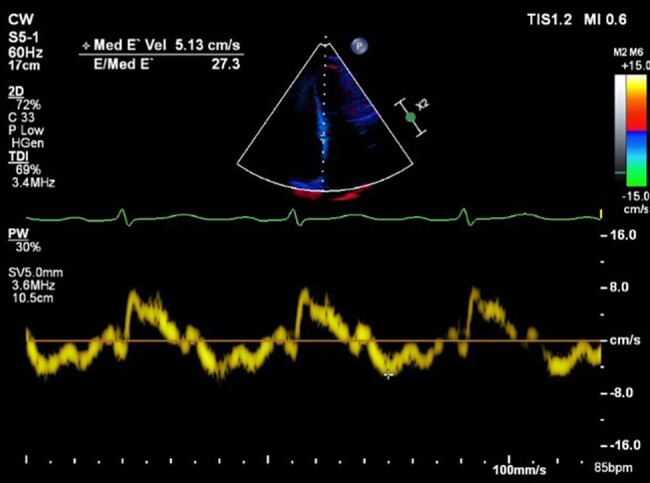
Abnormal medial E/e′ velocities (27.3) (tissue Doppler during transthoracic echocardiogram).
